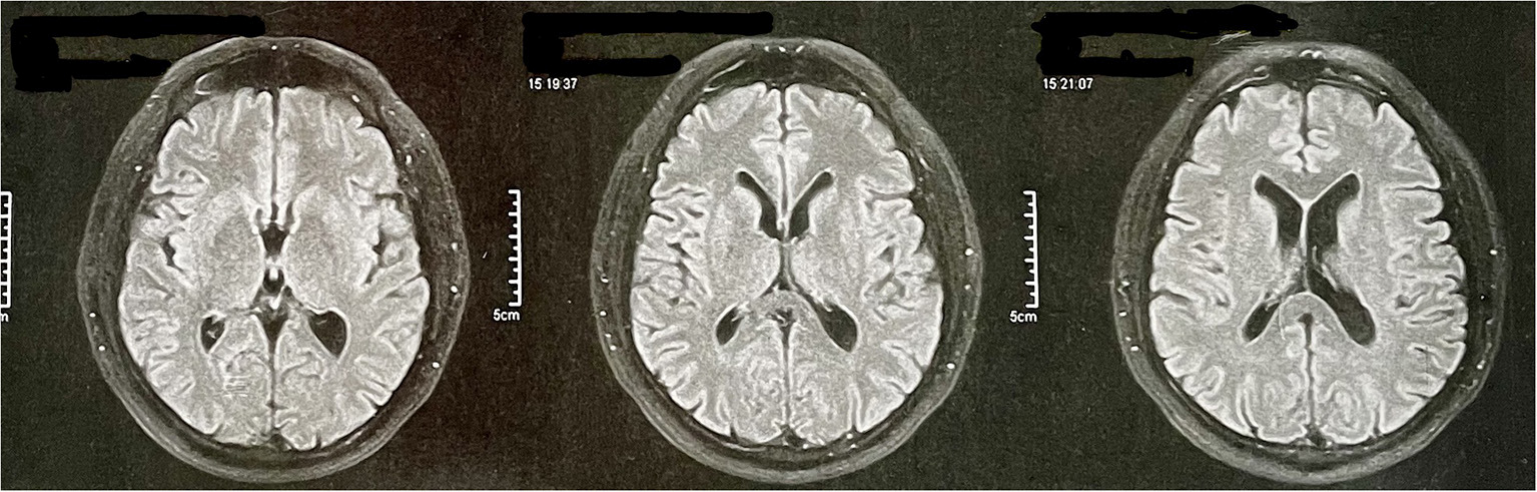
Patient's magnetic resonance angiography data. The magnetic resonance images are restricted to the T2 FLAIR sequence and only axial because they are derived from an angiographic resonance.
